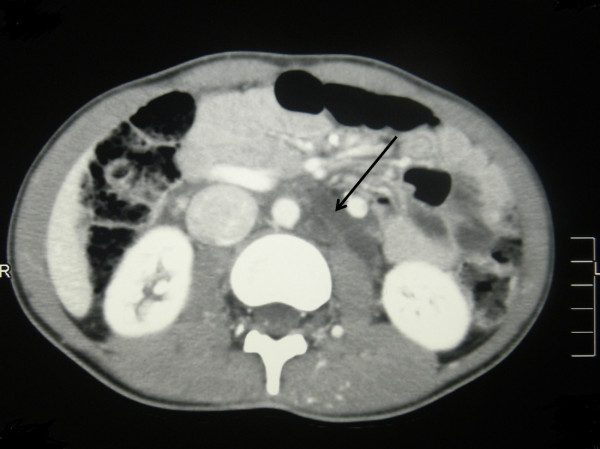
Contrast enhanced computed tomography of the abdomen showing the retroperitoneal lymphangioma (see arrow).
